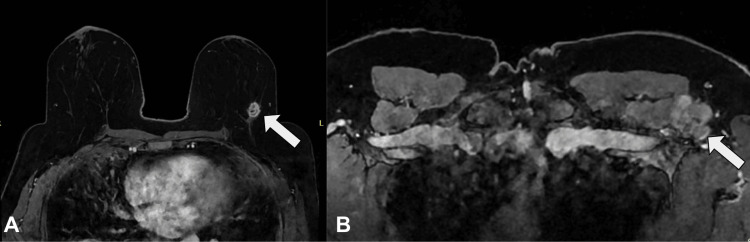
Pre-neoadjuvant breast and axilla MRI. (A) Breast MRI showing an avidly enhancing spiculated mass in the upper outer quadrant at the 2 o’clock position (arrowhead). (B) Axillary MRI showing an irregular mass with invasion of the pectoralis minor muscle (arrowhead).
